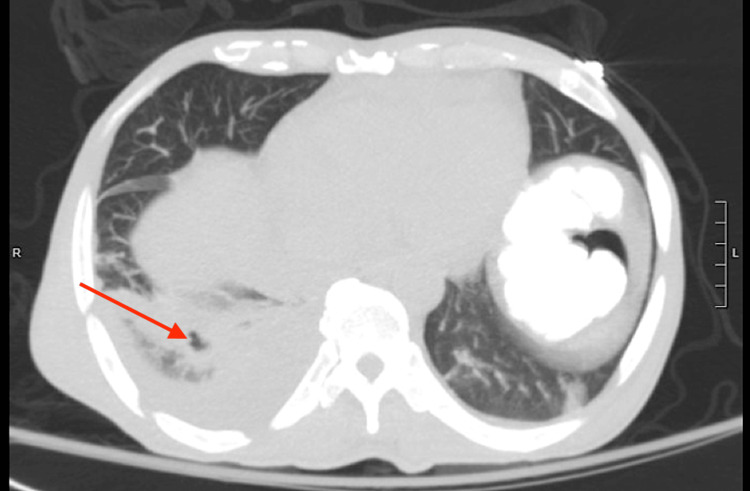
CT of the chest without contrast on hospital day 16 demonstrating new right lower lobe cavitation.
